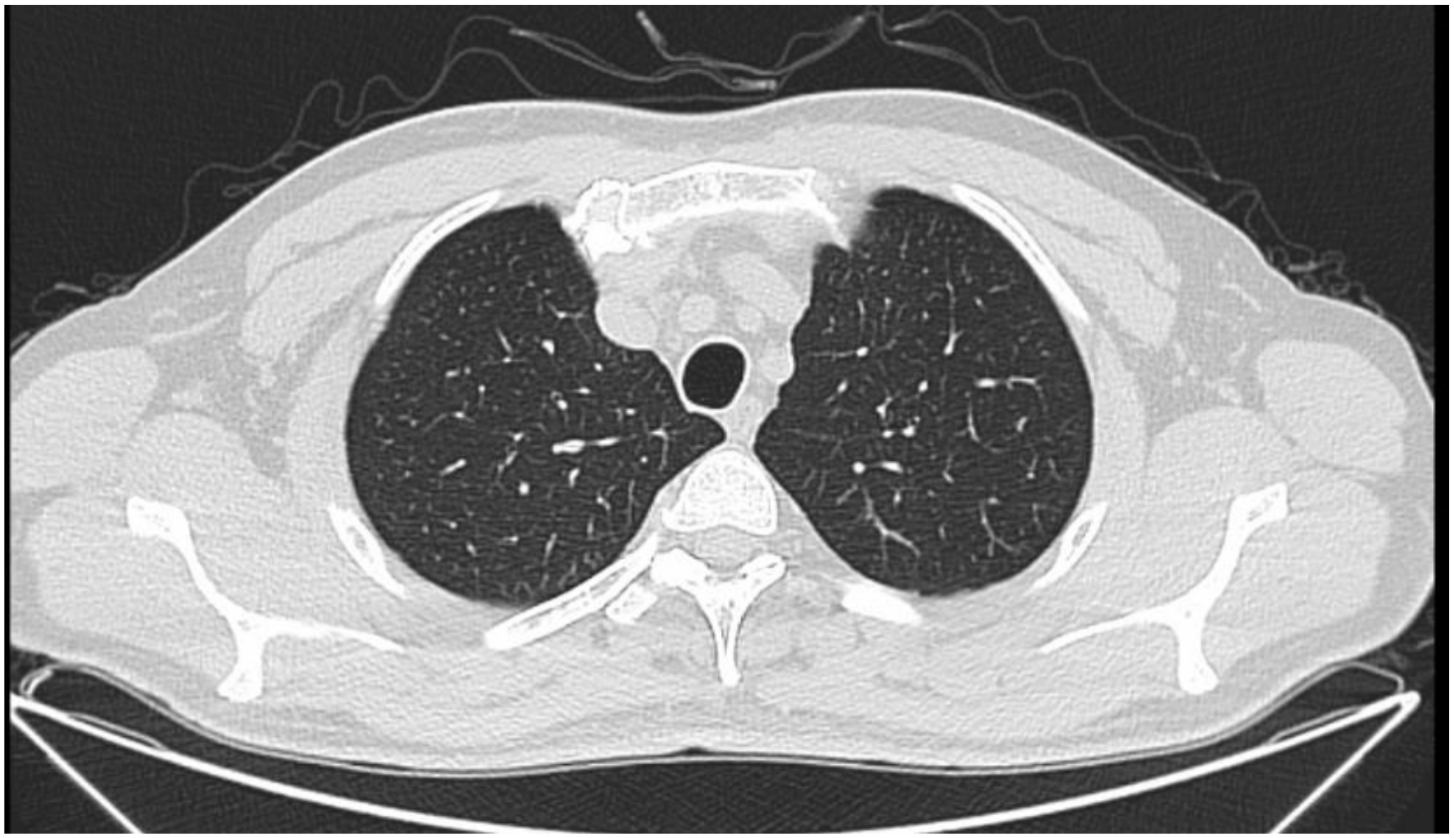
Postoperative Chest CT Scan: Chest CT imagine three months after removal of tracheal tumor: no significant thickening or infiltration of the trachea.
